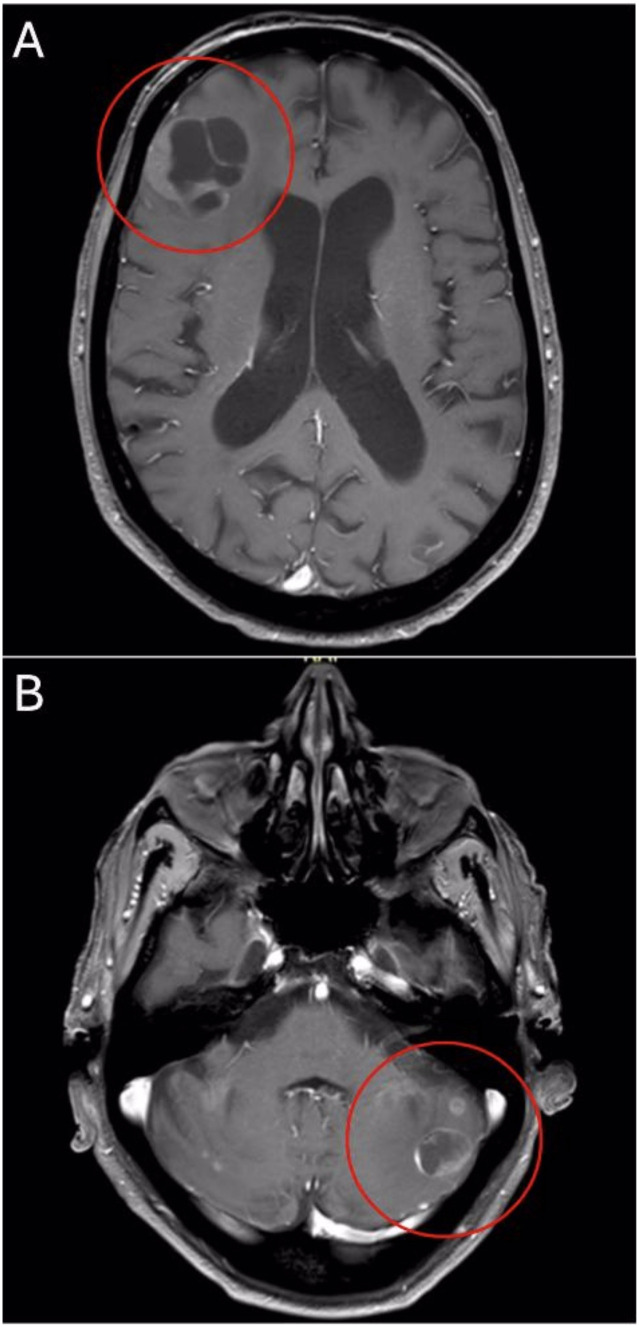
Axial T1-weighted MRI shows right frontal (A) and left cerebellar (B) lesions at primary diagnosis.
